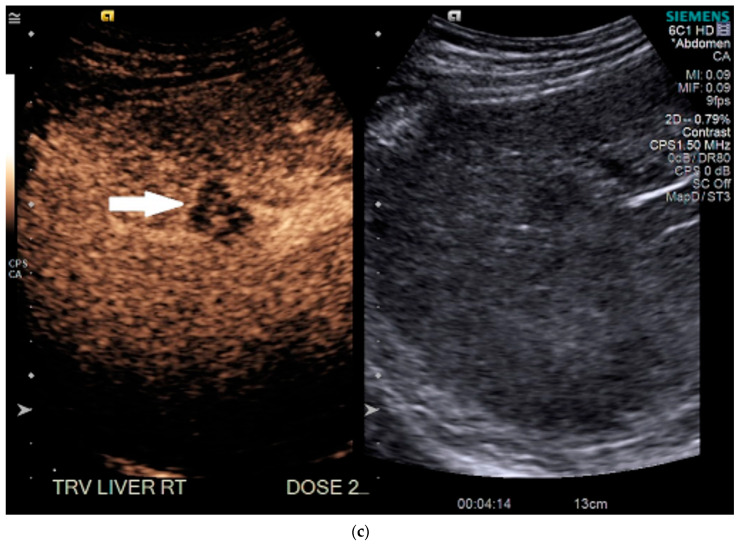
Patient 1 ultrasound images, with liver lesion denoted by white arrow: (a) grayscale images showing the hypoechoic lesion. Post contrast administration ultrasound in arterial phase with matched low-mechanical-index B-mode grayscale on the right and subtraction-type post contrast images on the left (b) and delayed phase (c) of the lesion, which shows marked washout. Liver Reporting and Data System (LI-RADS M).
